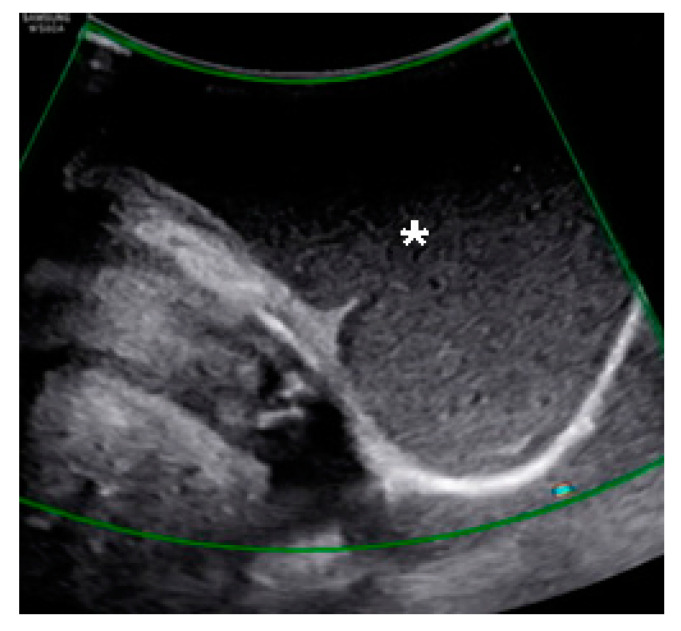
Trans-labial sonography showing a homogeneous hypoechoic semisolid cyst (asterisk) with a “ground-glass” appearance with no flow on the color Doppler imaging.
